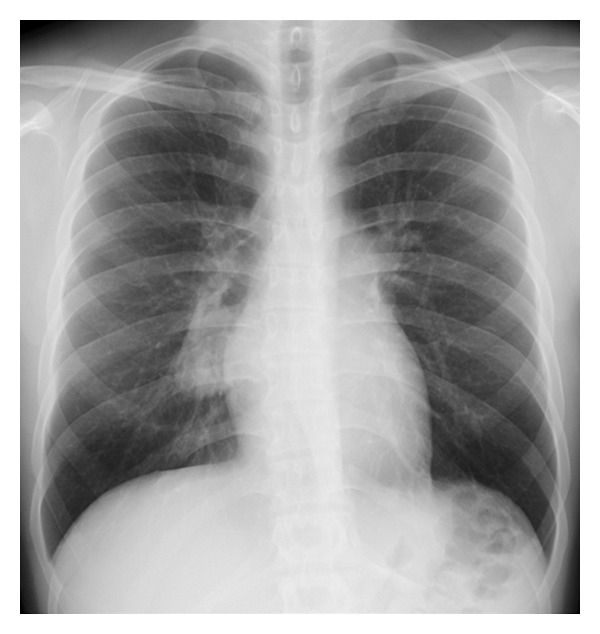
Chest radiograph shows a mass lesion located in the right infrahilar region.
